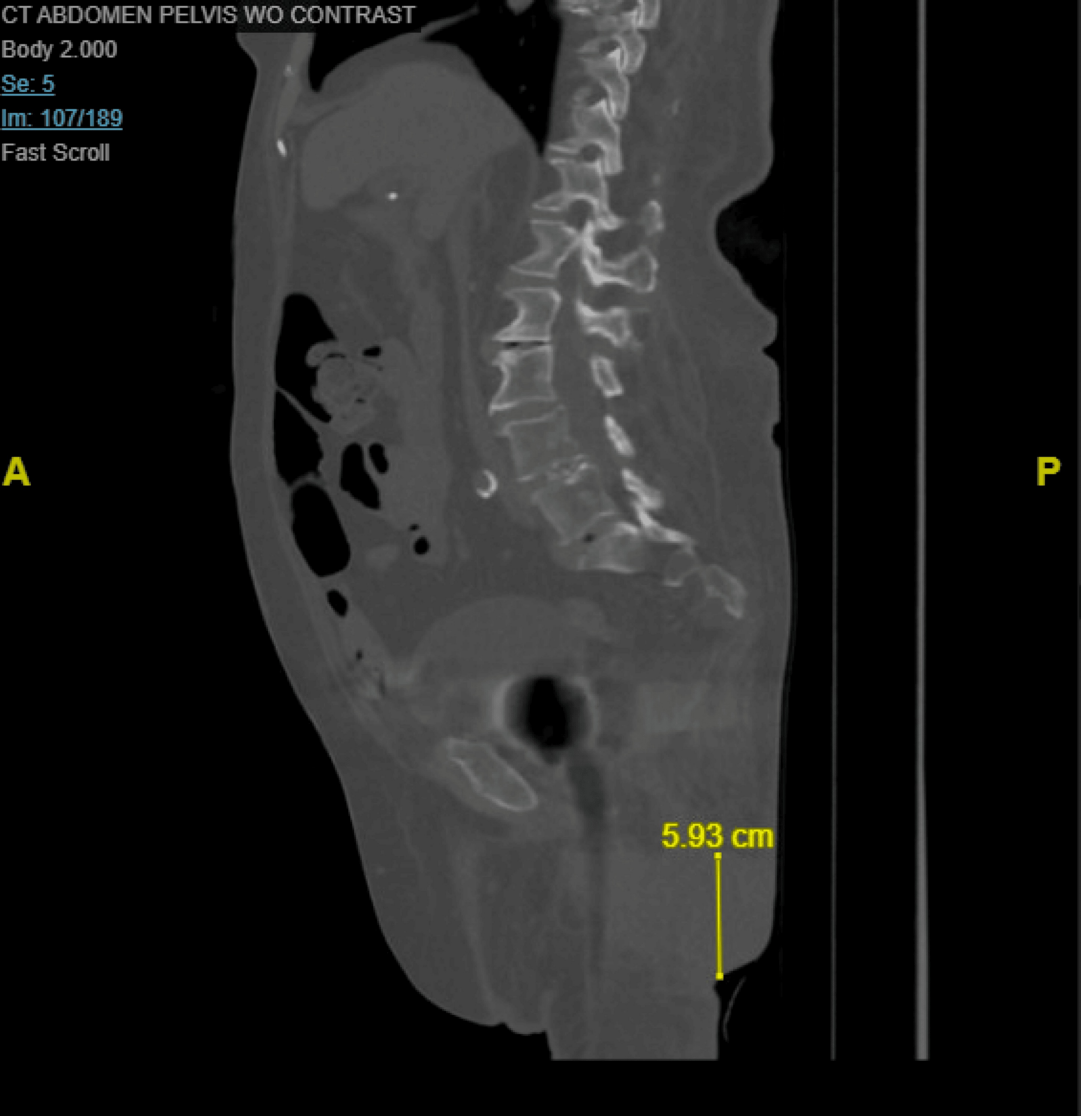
Sagittal non-contrast CT of the abdomen and pelvis Image demonstrating a fluid collection in the left gluteal region measuring approximately 5.93 cm in length, consistent with a gluteal abscess.
